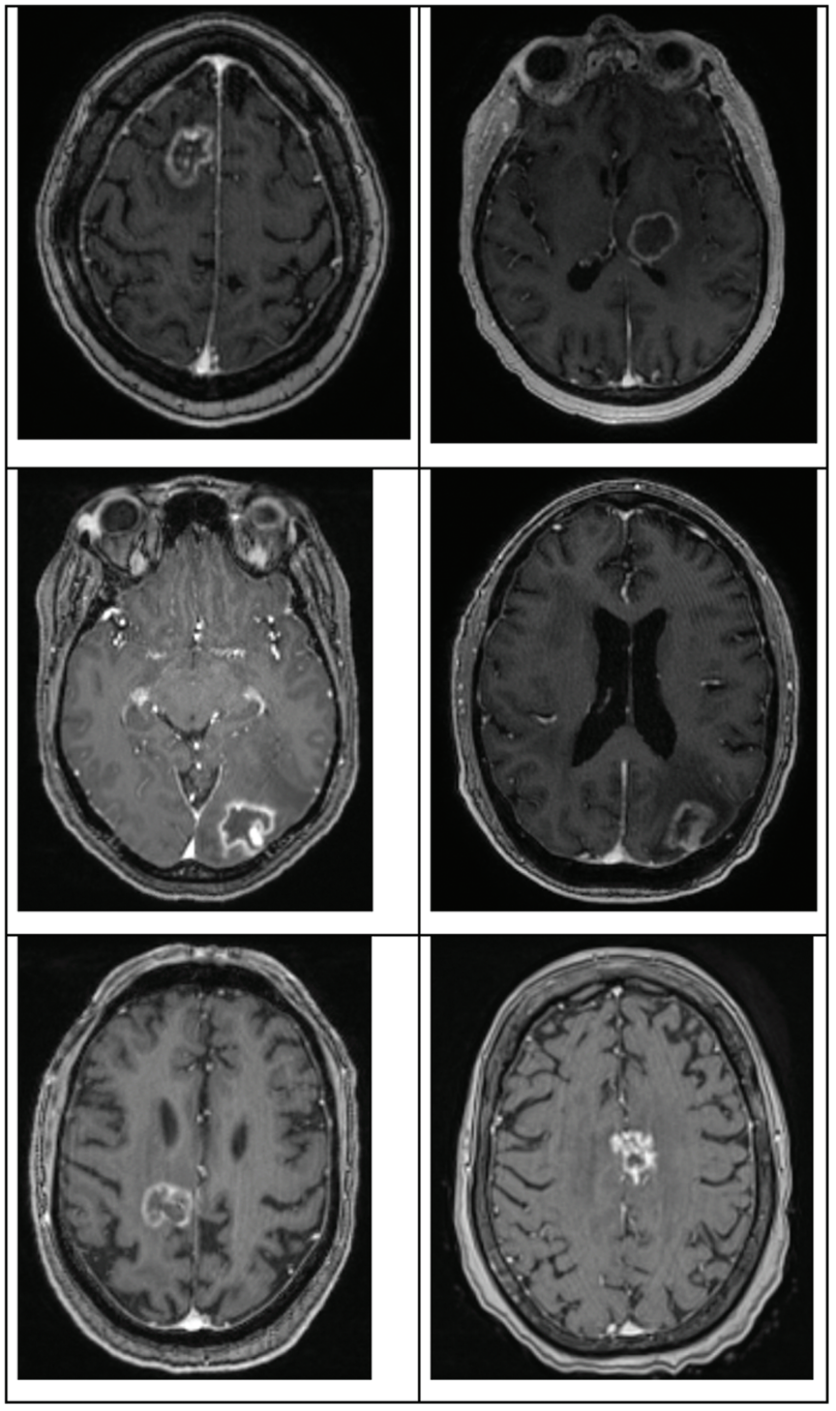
Clinical T1-weighted magnetic resonance imaging with contrast of radiographically progressive poststereotactic radiosurgery metastatic lesions in human patients. Each was subsequently treated with LITT and lesions on the left were proven to be radiation necrosis while lesions on the right were recurrent metastatic disease. LITT, laser interstitial thermal therapy.
